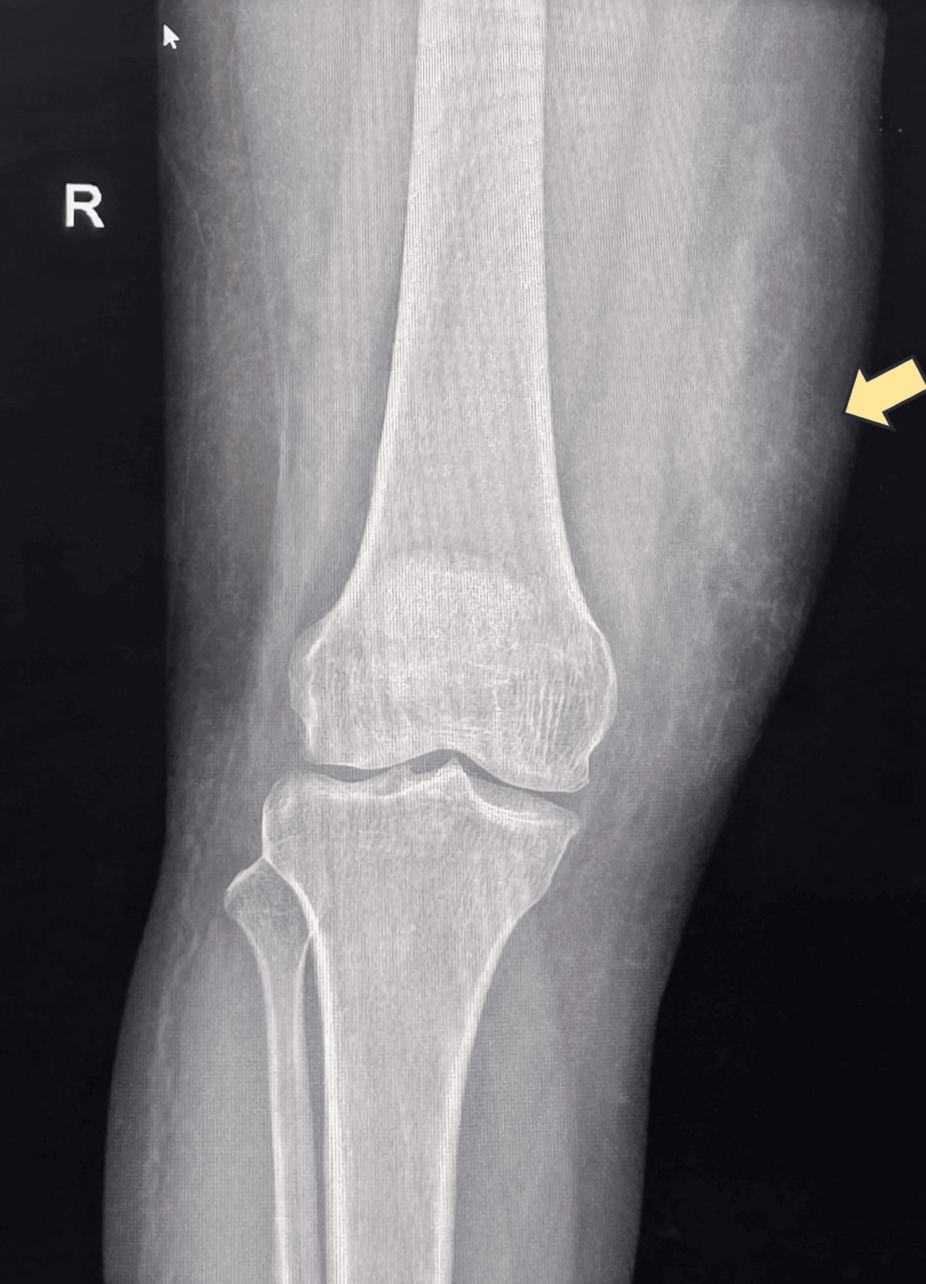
X-ray of the lower thigh, knee, and leg The arrow pointing to the Morel-Lavallée lesion.
